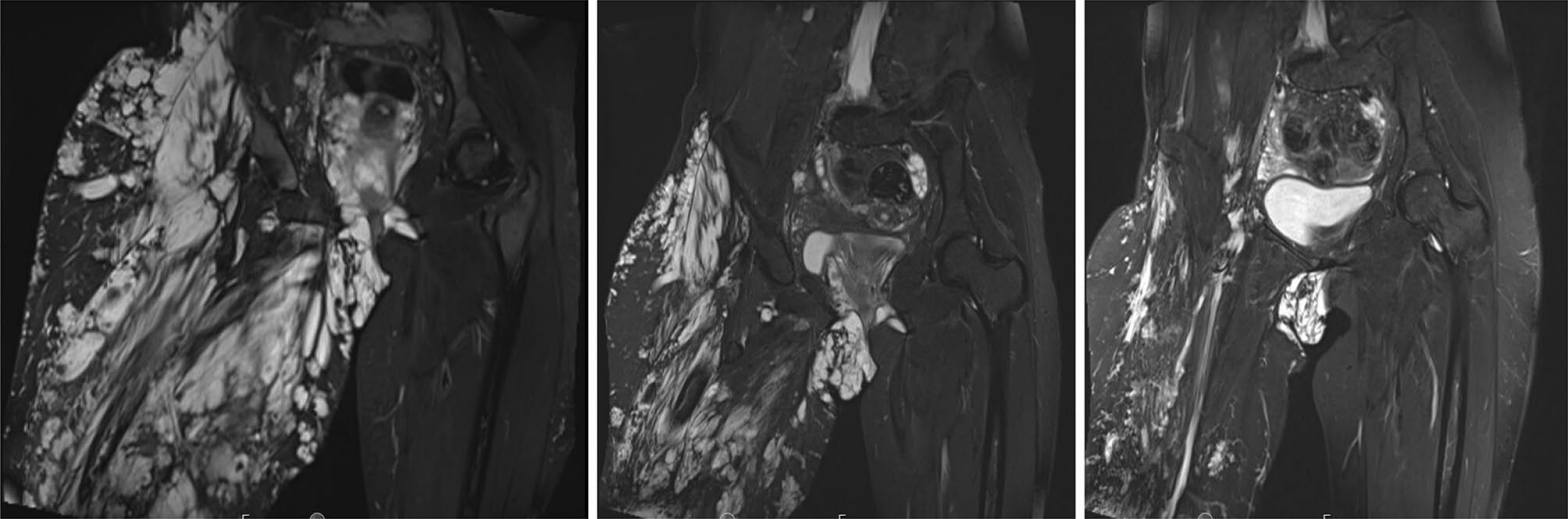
Patient number 2. MRI (T2 STIR cor. plane) of the pelvis and proximal thighs prior to (left), 6 months middle) and 12 months after alpelisib treatment (right).
